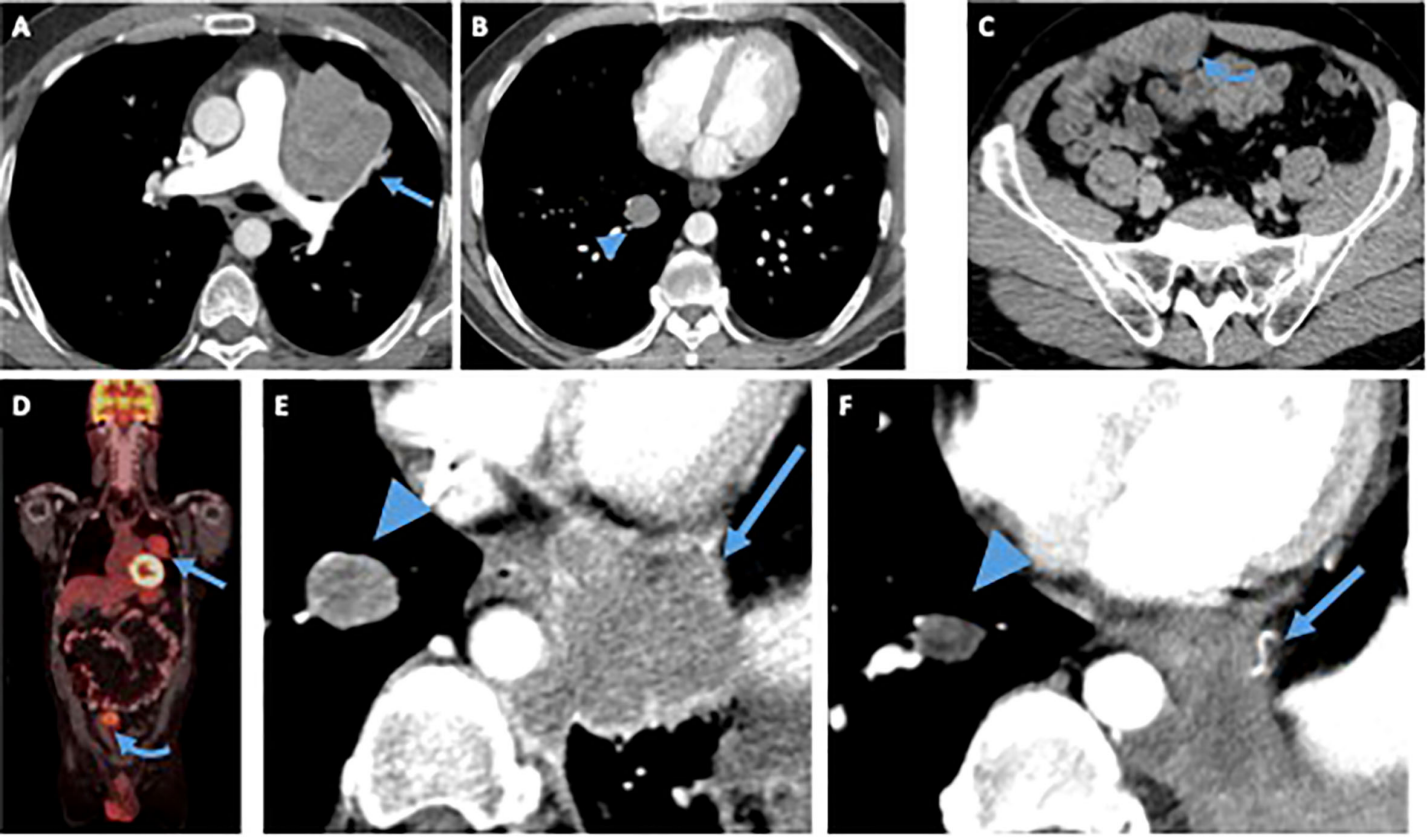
Patient 1. Contrast-enhanced computed tomography (CT) of the chest (A, B) abdomen and pelvis (C) at time of diagnosis demonstrates a left upper lobe paramediastinal mass (arrow), a right lower lobe lung nodule (arrowhead) and an enhancing solid right rectus sheath mass (curved arrow), fluorodeoxyglucose (FDG)-avid on a FDG positron emission tomography (PET)/CT (D) obtained at the same time. After treatment with vincristine/adriamycin/Ifosfamide, resection of the rectus sheath mass and radiation to the left lung mass, the patient started treatment with Pazopanib. Magnified view of an axial contrast-enhanced CT image of the chest (E) obtained before patient started treatment with Pazopanib shows the right lung nodule (arrowhead), and a new enhancing left paramediastinal mass (arrow), both showing mildly decreased size and enhancement on CT obtained 28 months after starting treatment (F), suggesting tumor regression.
